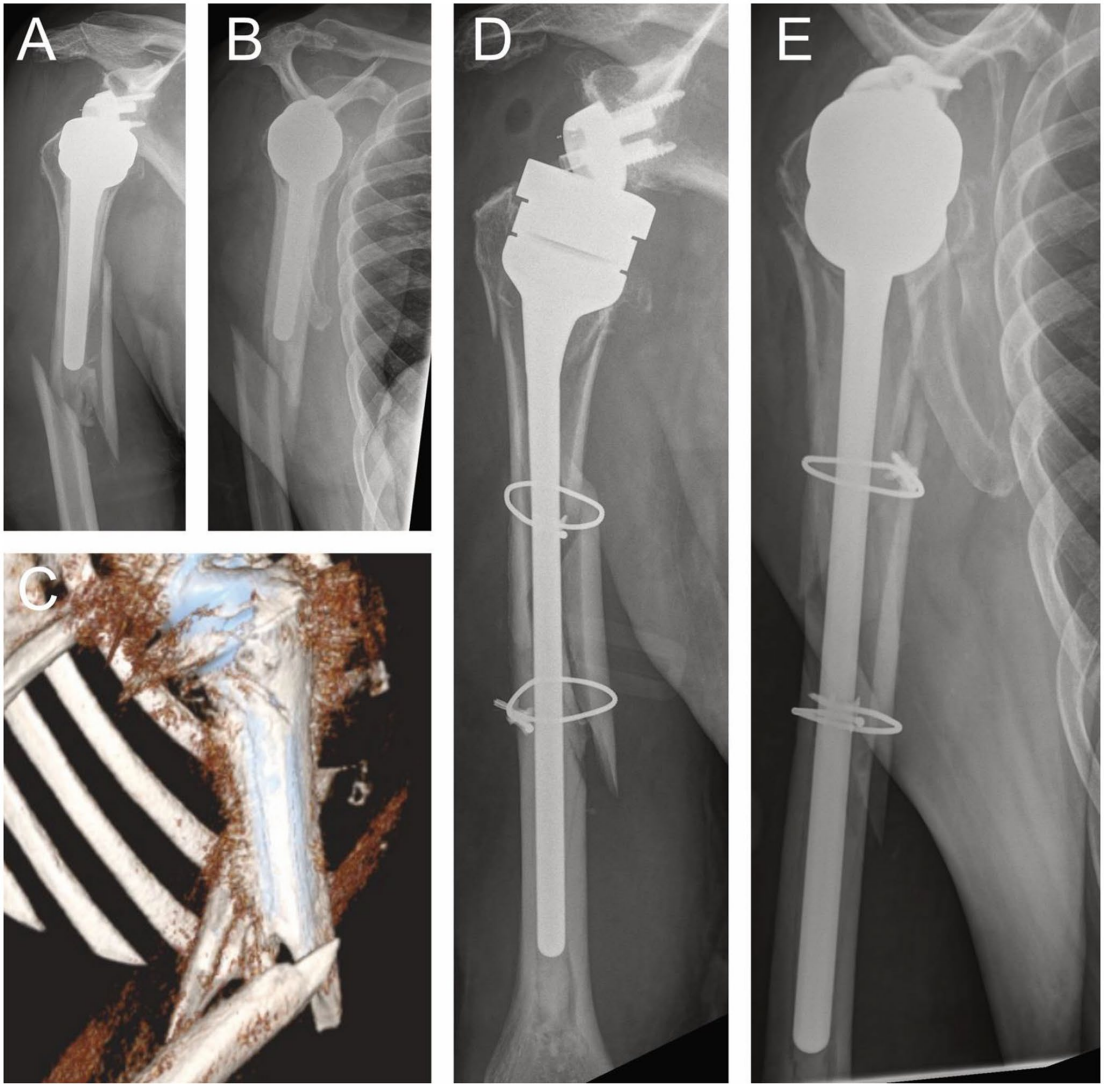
Preoperative a.p. and y-view X-ray with periprothetic fracture (A, B), 3D reconstruction in CT image auf the fracture C, and after surgical treatment with stem change inverse shoulder endoprosthesis a.p. X-ray and y-view X-ray (D, E)
